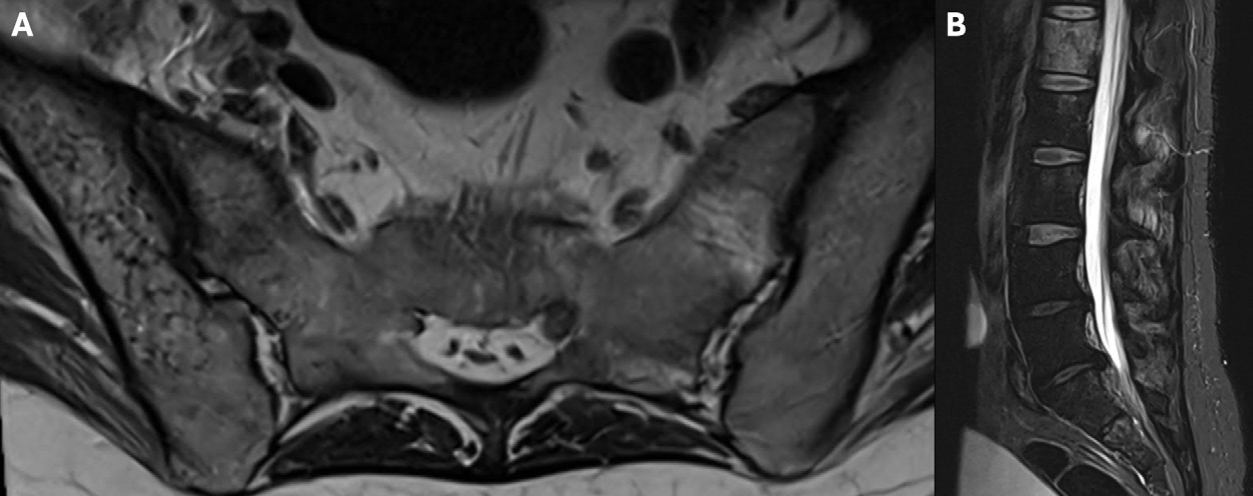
Axial T2-weighted (A) and sagittal STIR (B) MRI sequences confirm the presence of multiple hypointense foci in keeping with intraosseous gas within the right iliac wing and the L1 vertebral body. The L1 vertebral body also demonstrates diffuse increased signal compared with adjacent vertebrae, compatible with bone marrow oedema.
